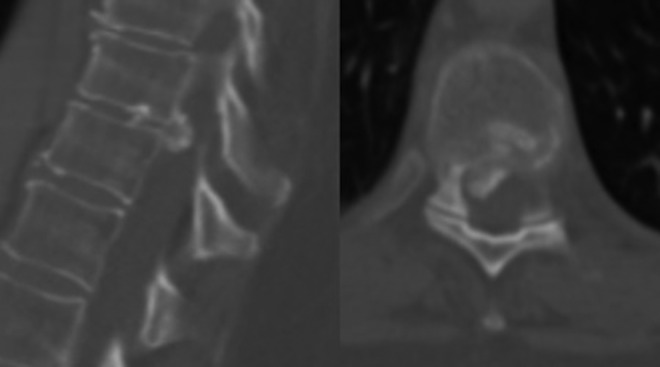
Midsagittal and associated axial computed tomographic images demonstrating a predominantly right-sided calcified disc at T9-10 spinal level with occlusion of the canal.
